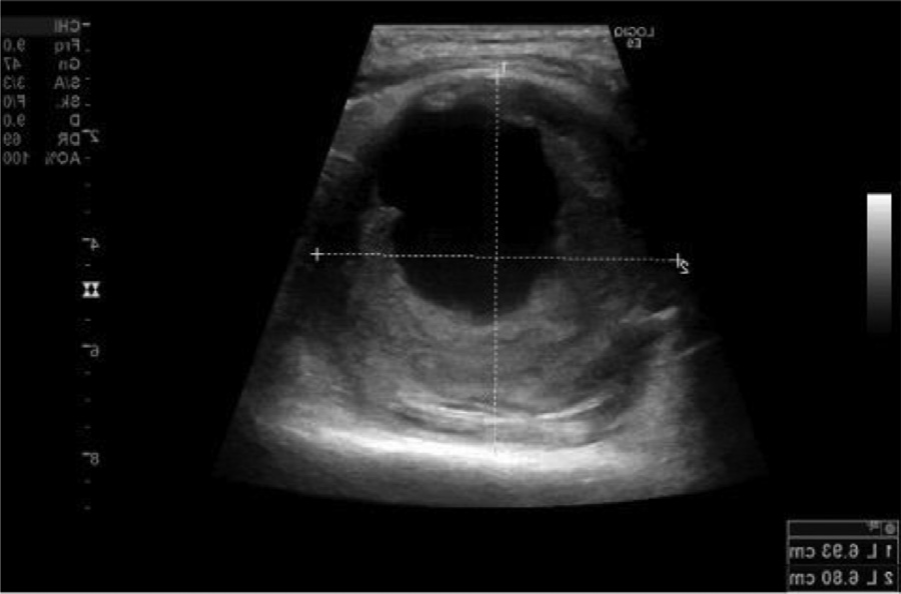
Ultrasonography of the popliteal artery. Legend 3: Hematoma with marginal thrombotic formations, size measurement of the pseudoaneurysm (6.8 × 6 cm).
